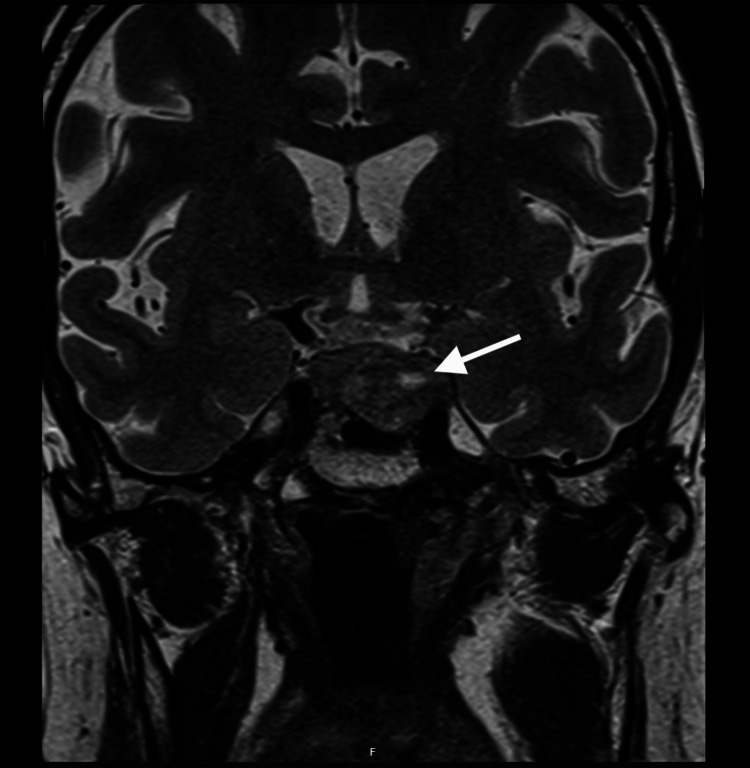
T2-weighted magnetic resonance imaging The image reveals an enlarged adenoma with a heterogeneous appearance (white arrow), containing mixed areas of hyperintensity and hypointensity suggestive of intralesional hemorrhage and/or necrosis.
